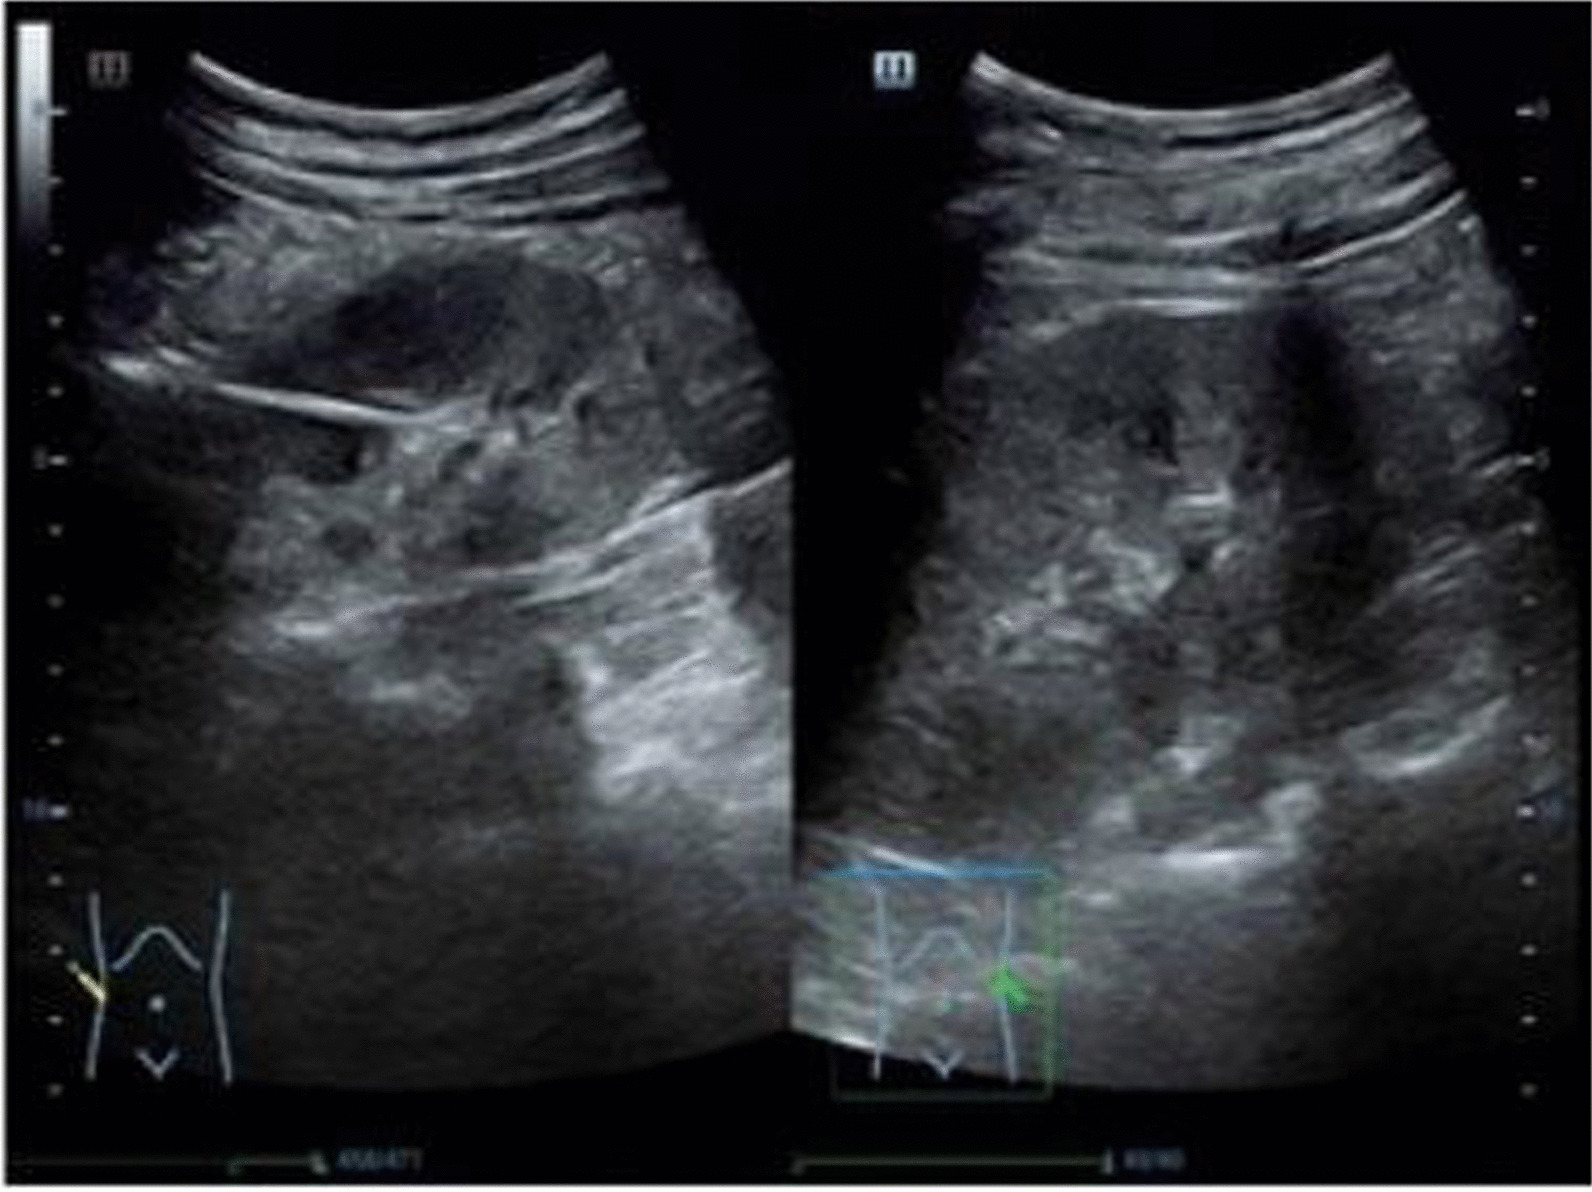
Strong stripe echo in the middle part of the right kidney was identified on ultrasound of the urinary system
